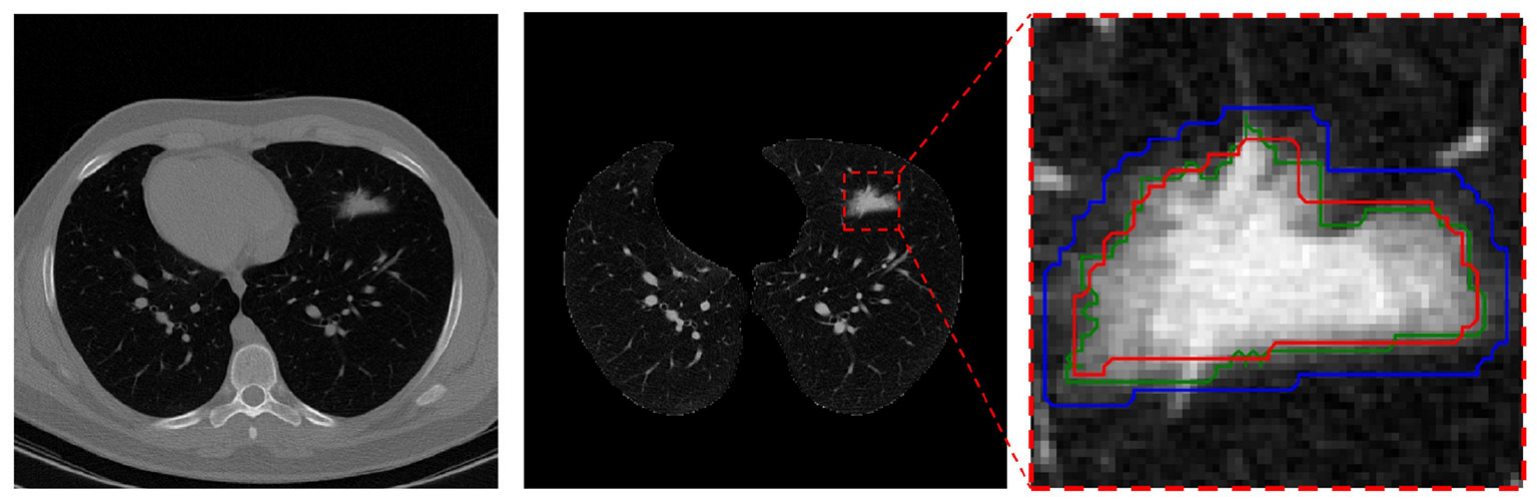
Mask pre-processing in the infection segmentation dataset. Left is the raw CT scan slice, middle is the lung image in the same slice (with infection in the upper-left lobe), and right is the segmented infection area in the image. In the right, the red contour depicts the radiologist manual mask edge, the blue contour is the dilated red contour, and the green contour s the rectified mask edge.
